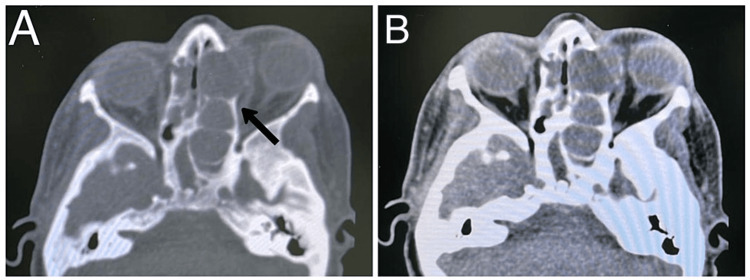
Case 7. Fronto-ethmoidal mucocele with proptosis, diplopia, and headache. Axial CT scan bone window at level of fronto-ethmoidal area showing expansile lesion occupying of the left anterior ethmoid (arrow), and left frontal with lateral displacement of lamina papyracea.
